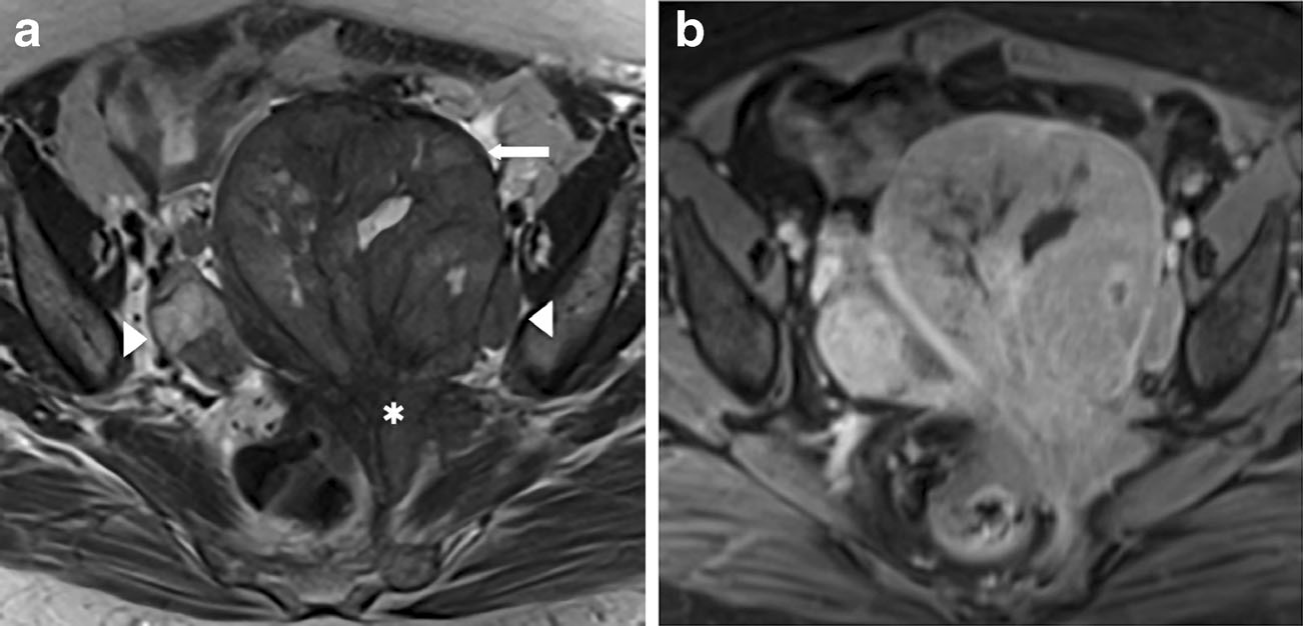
a–b Cervical small cell carcinoma (FIGO III-B; extension to the pelvic side wall). A 64 year-old female complaining of menorrhagia. Axial T2-weighted MR image (a) and axial T1-weighted MR image with fat saturation after intravenous gadolinium administration (b). Cervical tumour extending posteriorly to the pelvic wall (*asterisk*) and superiorly to the left adnexal area, where it forms a huge solid, heterogeneous mass with central necrotic areas (*arrow*). This mass shows intense enhancement after gadolinium administration and is adherent to the uterine corpus, which may be found inferiorly. Bilateral internal iliac lymph nodes are also noted (*arrowheads*)
